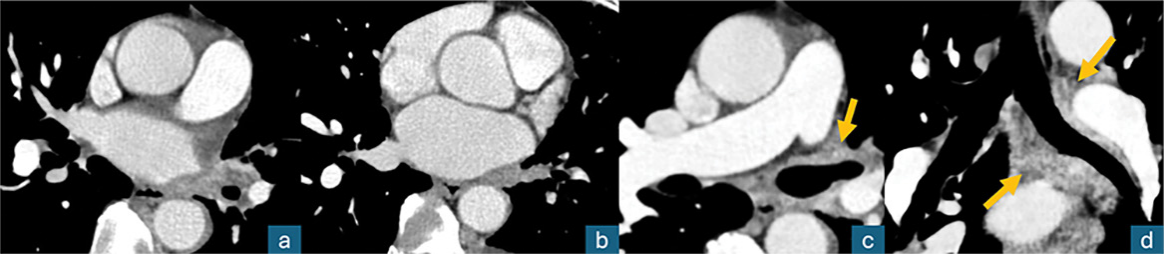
Contrast‑enhanced axial chest CT (mediastinal window); a, b: thrombosis of the left superior and inferior pulmonary veins; c, d: diffuse left peribronchial contrast‑enhancing infiltration (arrows), “pulmonary hilar cavernoma.”
